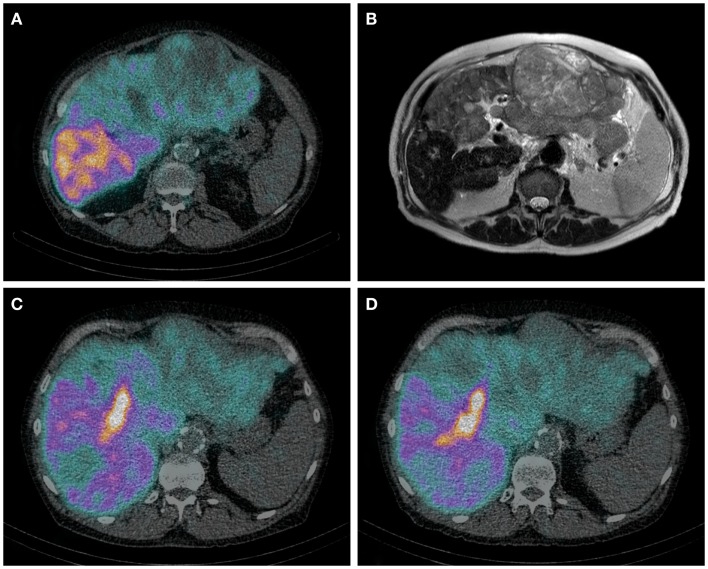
(A–D) Axial HBS SPECT-CT image through the abdomen of a patient with hemochromatosis and multifocal HCC. Notice the regional uptake differences in cirrhotic and tumorous tissue (A). Corresponding MRI T2 weighted image (B). Same patient before (C) and after (D) RE of the left lobe including segment 4. (C) It is from the same HBS SPECT/CT as image (A), but shown in a different axial plane. The decrease of ^99m^Tc-mebrofenin uptake after treatment is best visible in segment 4. The area of high uptake is biliary excretion in a dilated bile duct.
